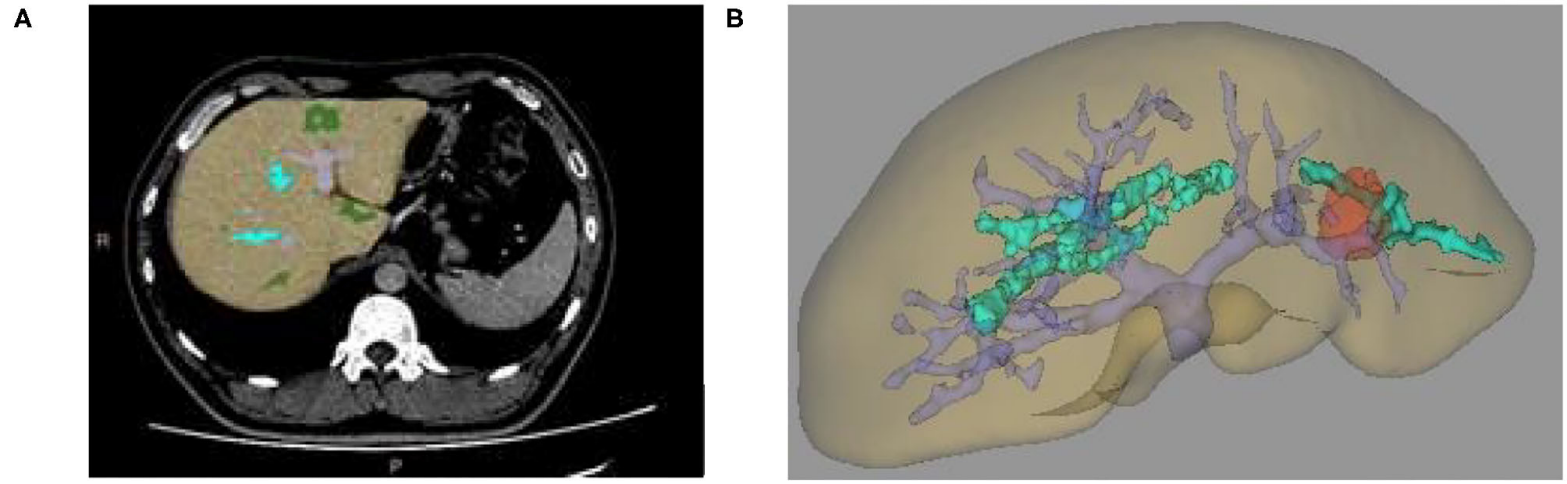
(A) Two-dimensional CT enhanced image, (B) Three-dimensional (3D) reconstruction of liver and tumor based on growth model on CT data.
